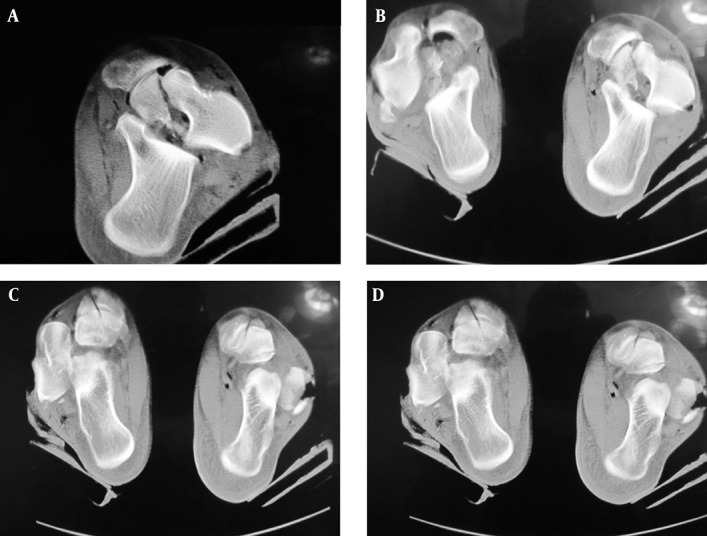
Ankle CT Scan of The Patient With Bilateral Talar Fracture-Dislocation
